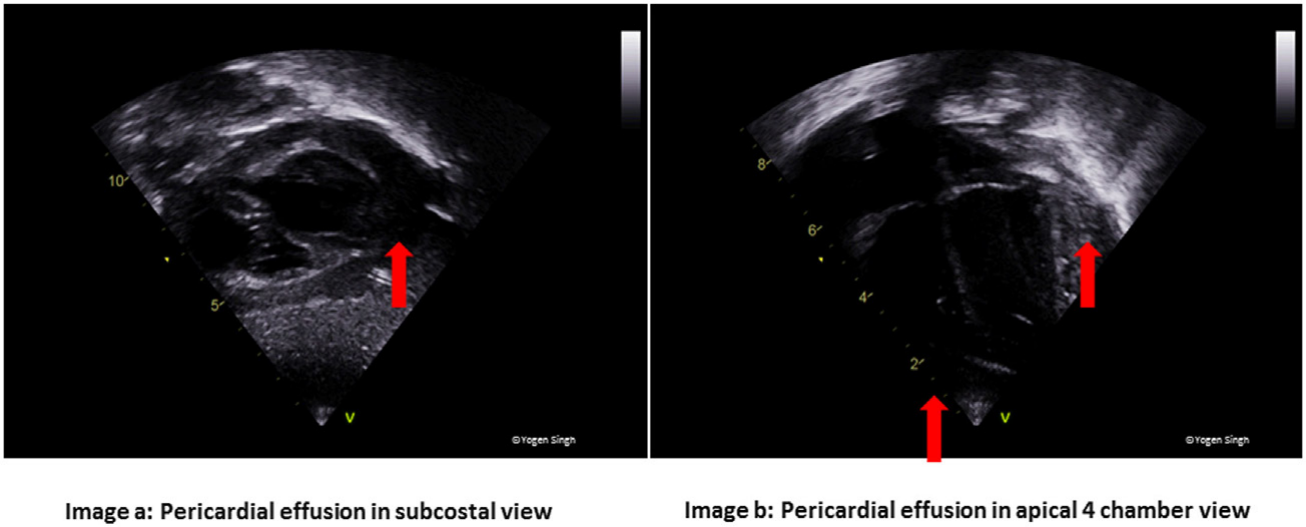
Pericardial effusion in (A) subcostal and (B) apical 4 chamber views. In large pericardial effusion and cardiac tamponade, there may be collapse of cardiac chambers—first seen collapse of right atrium followed by right ventricle.
